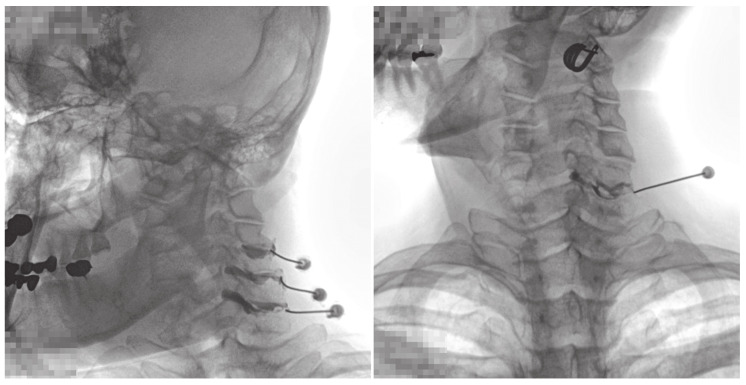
Arthrogram X-ray images showing extra-cervical facet joint flow.
